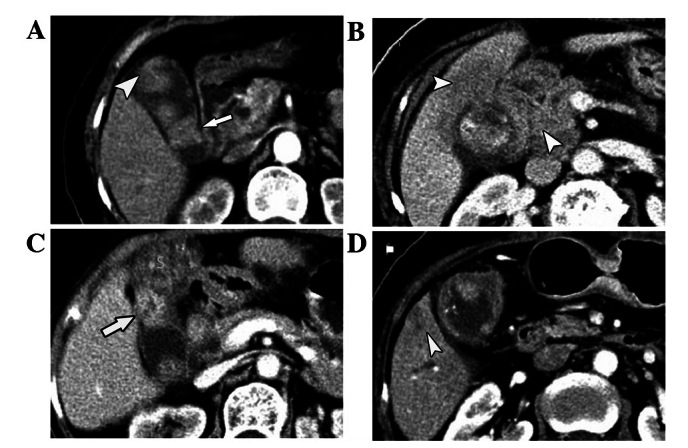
Computed tomography (CT) images of gallbladder cancer. In all cases the tumors were solid, the base of the tumor was wide and the demarcation between the tumor and wall was obscure. (A) Arrowhead and arrow show multiple tumors with wide base; the white arrow shows the tumor-infiltrated and contracted wall. (B) Gallbladder cancer which infiltrated the neighboring tissue (white arrowhead). The pathological diagnosis was obtained by biopsy. (C) White arrow indicates the infiltrated wall and the disappearance of the space between the wall and liver. (D) Arrowhead indicates the metastasis in the liver parenchyma.
